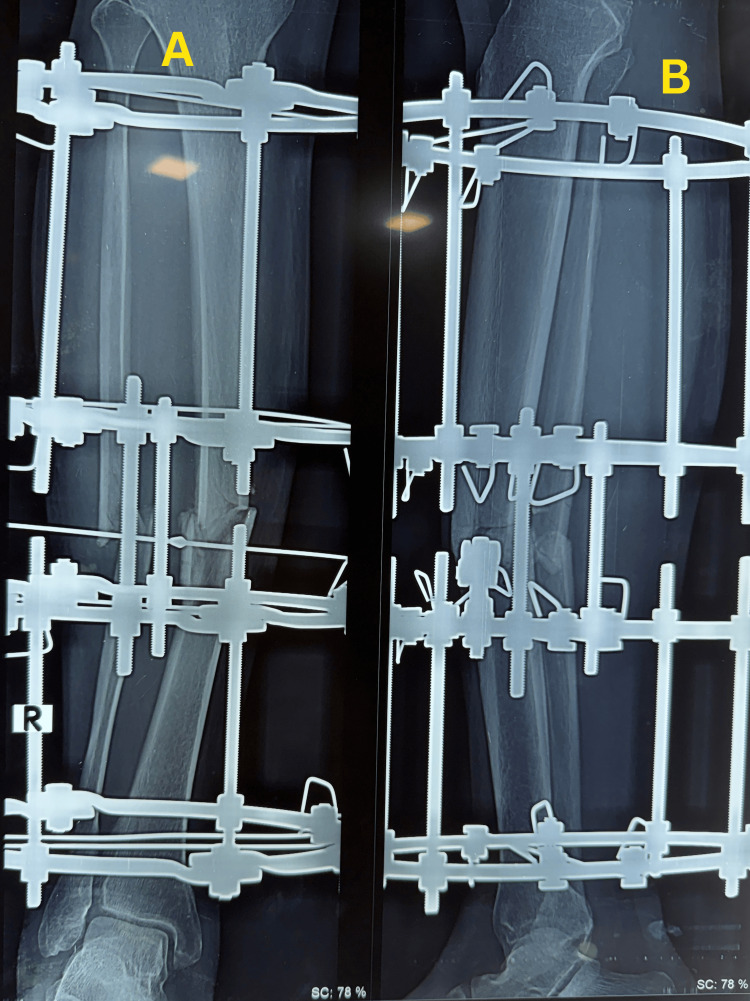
Follow-up radiographs demonstrating callus formation at the tibial fracture site (A) Anteroposterior view demonstrating progressive callus formation at the midshaft of the right tibia. (B) Lateral view showing maintained fracture alignment with developing callus across the fracture site.
